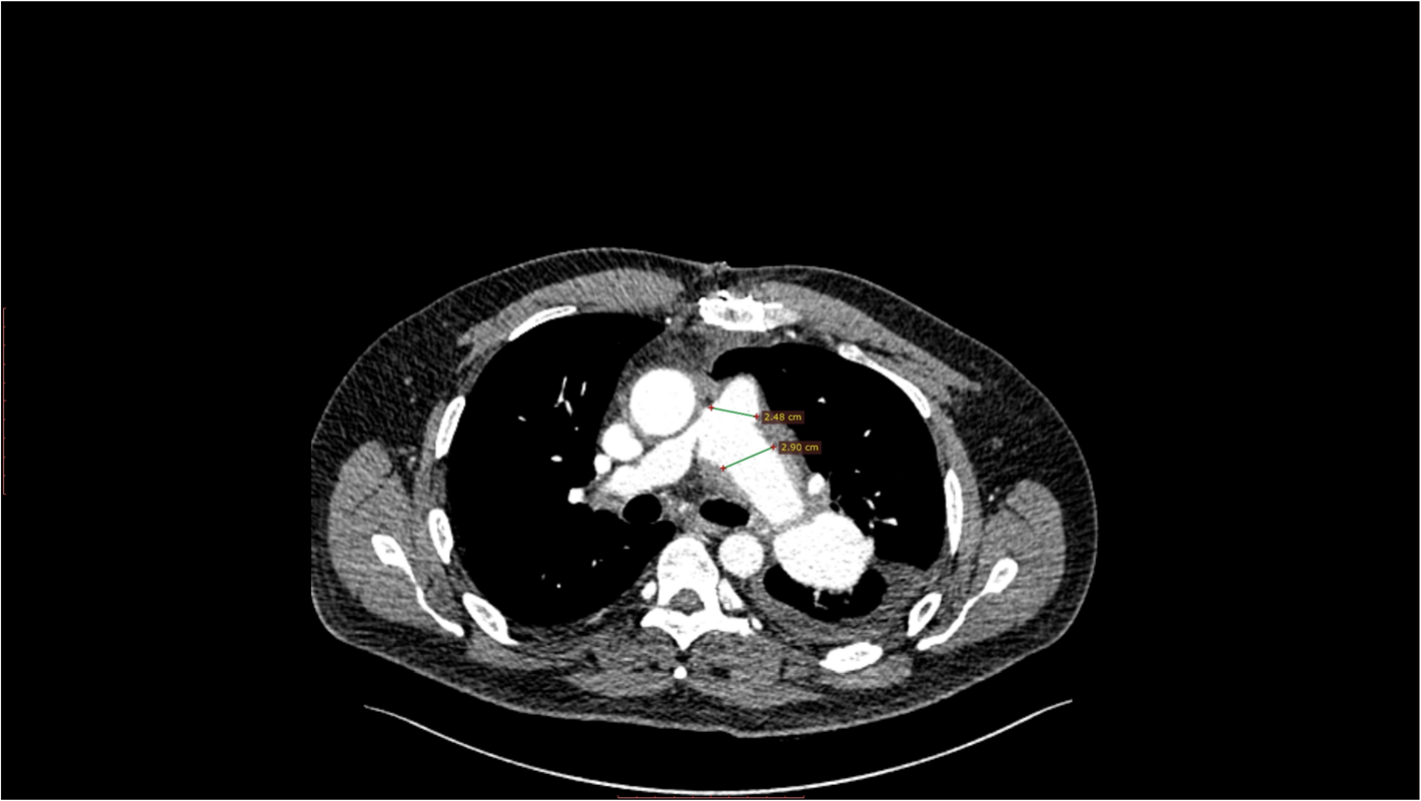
Chest computed tomography angiogram scan (CT) showing: Pulmonary artery trunk, left pulmonary artery and right pulmonary artery replaced by Wovex tube graft without dilatation in the great vessels or stenosis
